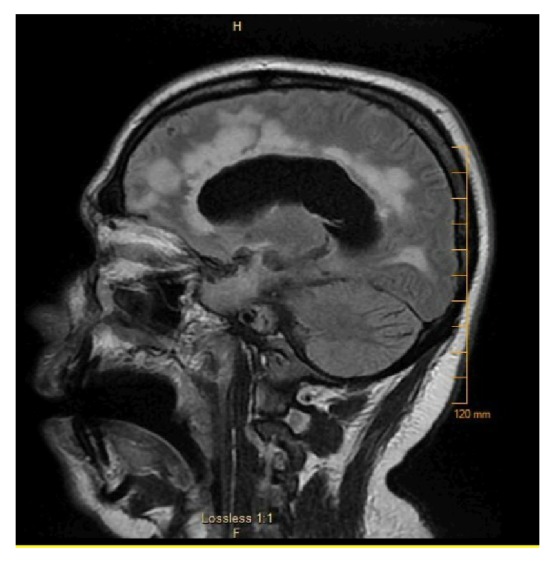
Post-cyclophosphamide MRI showed progressive periventricular, mid, and also a component of superficial/juxtacortical white matter T2/FLAIR hyperintensity, the latter of which is more apparent within the frontal lobes. There has been further progression of hydrocephalus with diffuse ventricular enlargement.
